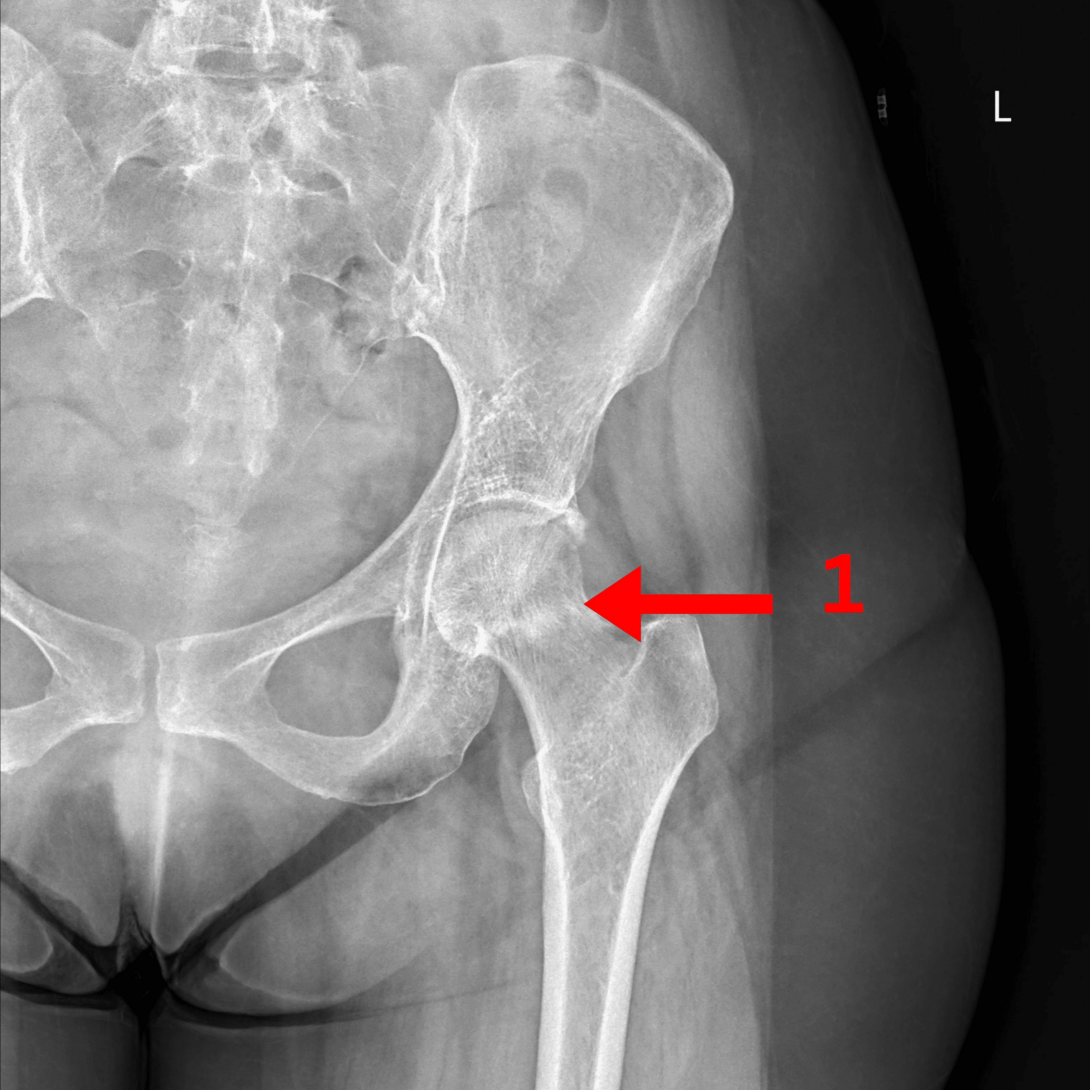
X-ray of the left hip showing (1) subcapital sclerotic fracture line
